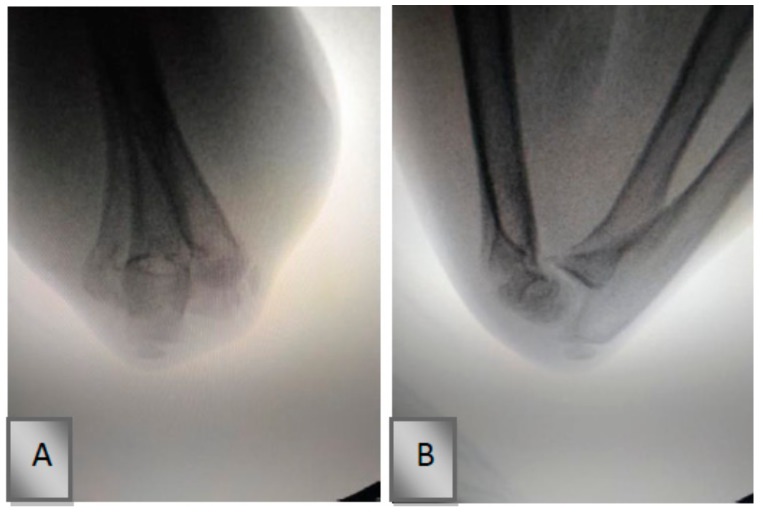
Fluoroscopic image of the supracondylar fracture after fixation with a fabric adhesive bandage. (A) Anteroposterior image and (B) lateral X-ray image of the left elbow.
